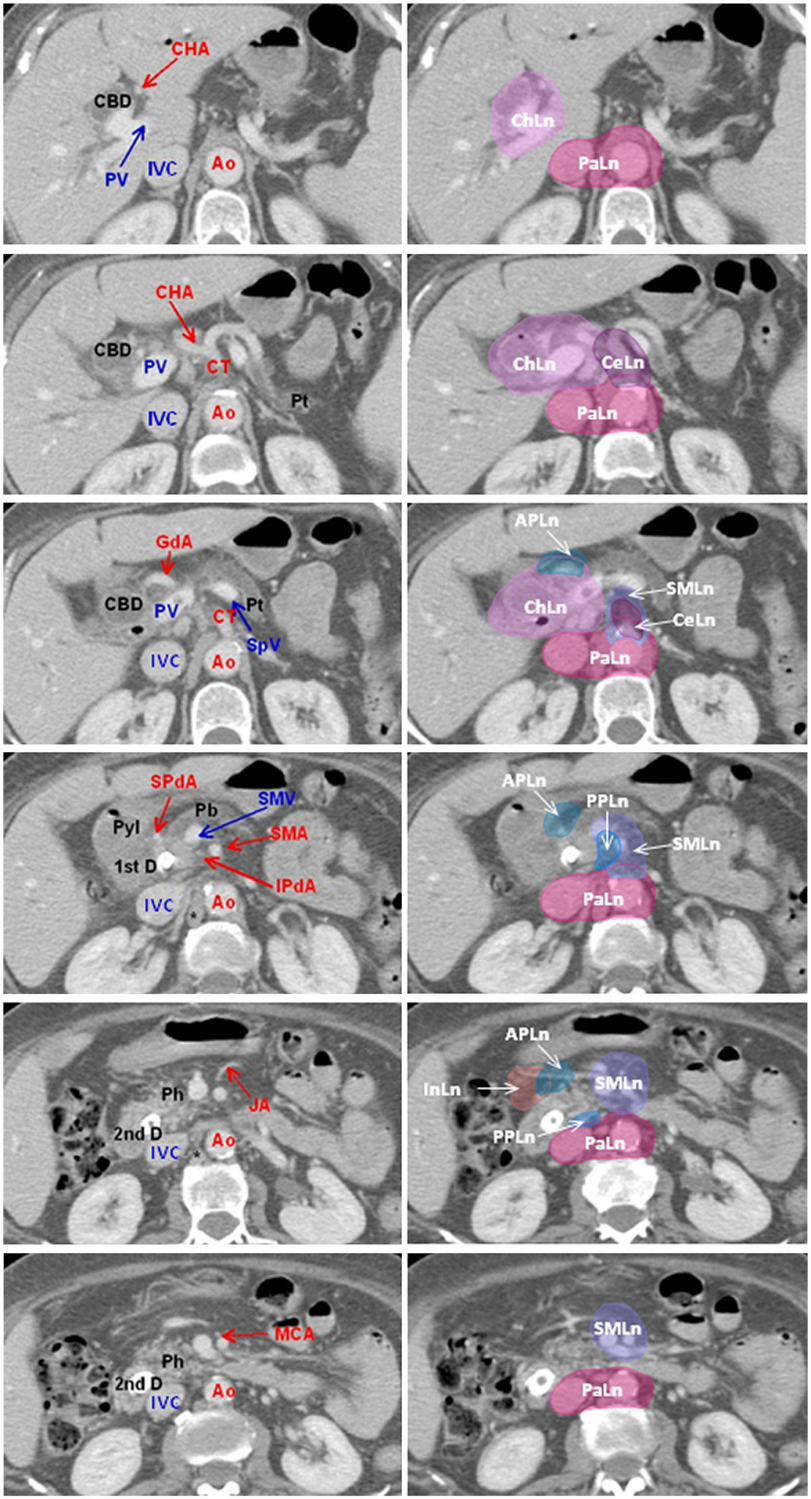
Anatomical structures for the high risk lymph nodal regions of the head pancreatic cancer. Transverse CT slices through high risk lymph node regions of the head pancreatic cancer from cranial to caudal direction. The abdominal artery and the anatomical structures were identified as a surrogate target for lymph node regions. A margin of 10 mm was added to the artery to delineate lymph node area, including the soft tissue with lymphatic and neural plexus. CTV was formed by the union of the identified lymph node areas. *Abbreviations*: Ao = Aorta; APLn = Anterior Pancreaticoduodenal Lymph nodes; CBD = Common Bile Duct; CeLn = Celiac Lymph nodes; CHA = Common Hepatic Artery; ChLn = Common hepatic and hepatoduodenal ligament Lymph nodes; CT = Celiac Trunk; 1st D = first part of Duodenum; GdA = Gastroduodenal Artery; InLn = Infrapyloric Llymph nodes; IPdA = Inferior Pancreaticoduodenal Artery; IVC = Inferior Vena Cava; JA = Jejunal Artery; MCA = Medial Colic Artery; PaLn = Paraaortic Lymph nodes; Pb = Pancreatic body; Ph = Pancreatic head; Pt = Pancreatic tail; Pyl = Pylorus; PPLn = Posterior Pancreaticoduodenal Lymph nodes; PV = Portal Vein; 2nd D = second part of Duodenum; SMA = Superior Mesenteric Artery; SMLn = Superior Mesenteric Lymph nodes; SMV = Superior Mesenteric Vein; SPdA = Superior Pancreaticoduodenal Artery; SpV = Splenic Vein; * = pillar of the diaphragm.
